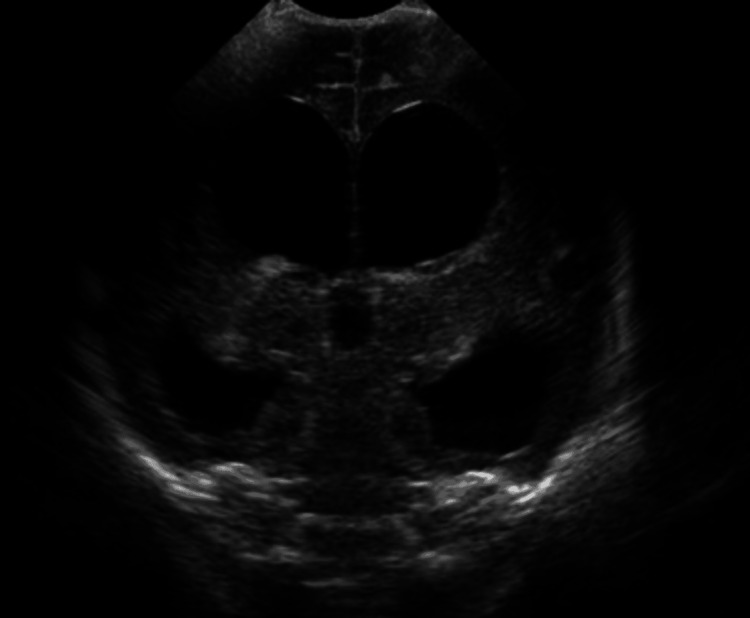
Neurosonogram shows dilatation of ventricular system Credit: Image taken by authors at the hospital.
